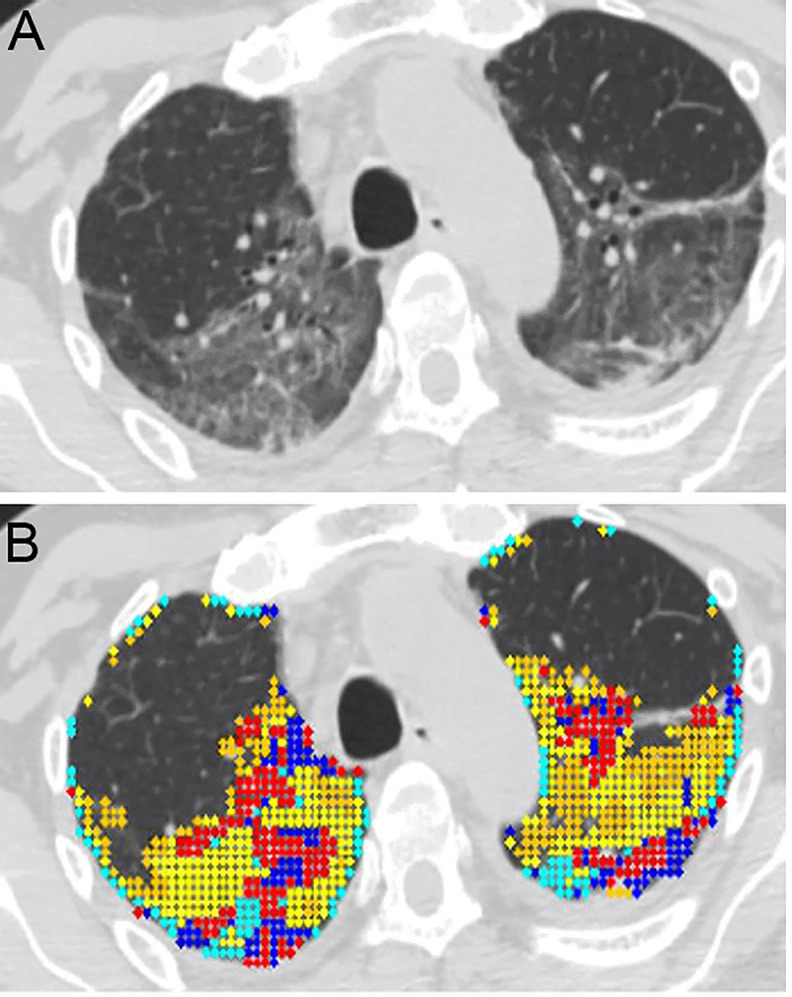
Quantitative high-resolution CT analysis of COVID-19 acute respiratory distress syndrome (ARDS). A. Horizontal high resolution (HR)-CT lung cut of a selected COVID-19 ARDS (CARDS) patient. The patient is a 61-year-old male with mandatory mechanical ventilation on arrival to the long-term-acute care facility.
